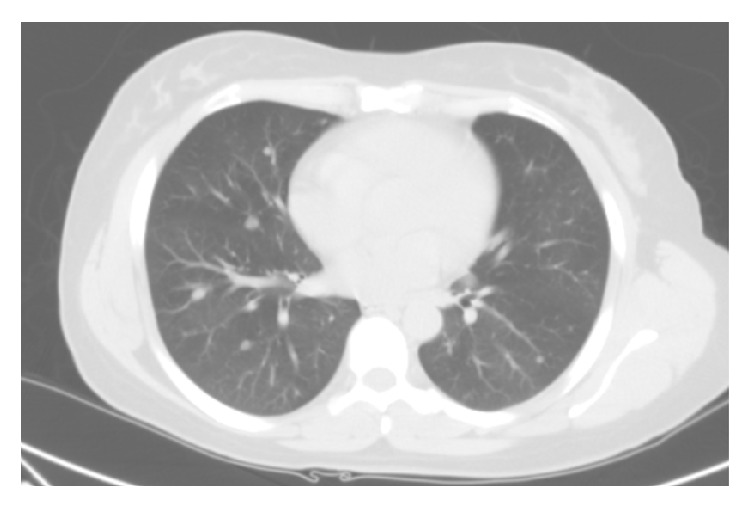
CT chest. Multiple subcentimeter bilateral lung nodules.
